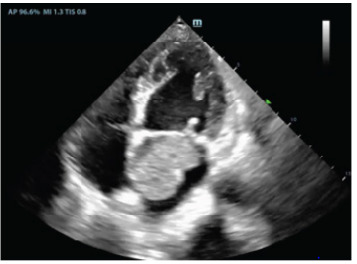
Computed tomography coronal view demonstrating a 3 x 3 x 5 centimeter left atrial mass (arrow) later confirmed to represent an atrial myxoma.
